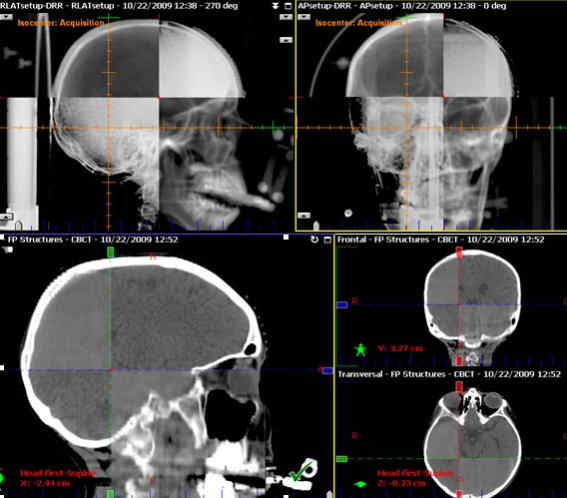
Comparison of kV‐DRR (top) and 3D‐CBCT (bottom) matching of a recently‐treated patient.
